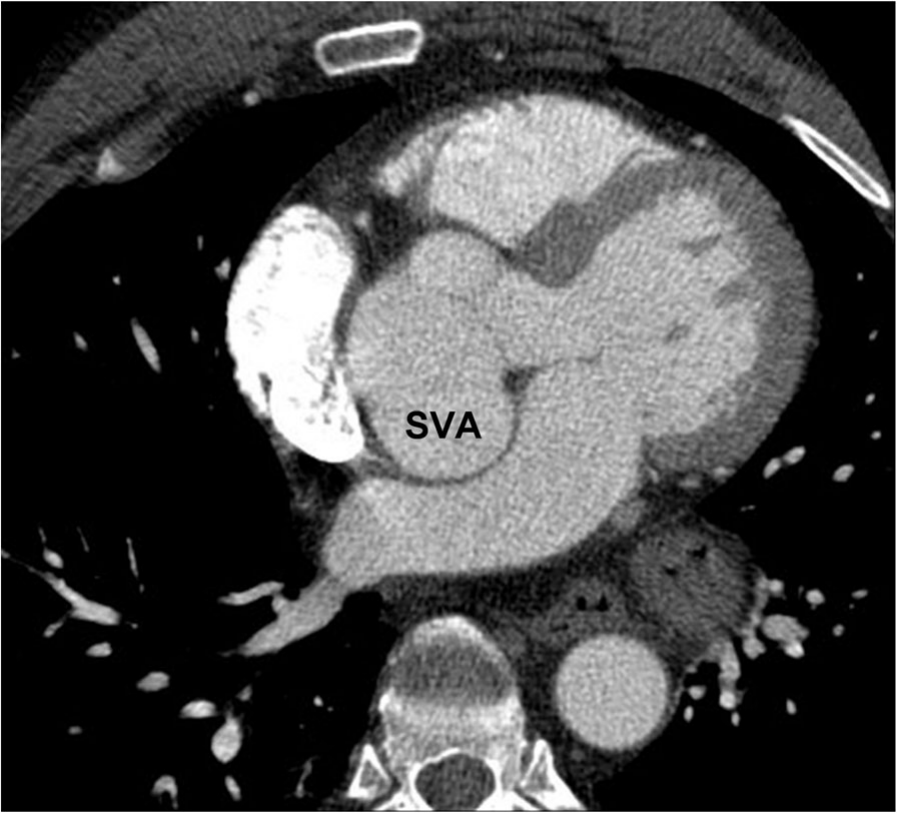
Sinus of Valsalva aneurysm (SOVA) of the non-coronary sinus on an axial contrast enhanced thoracic CT in a 59-year old male being evaluated for thoracic neoplasm. *SVA* sinus of Valsalva aneurysm
